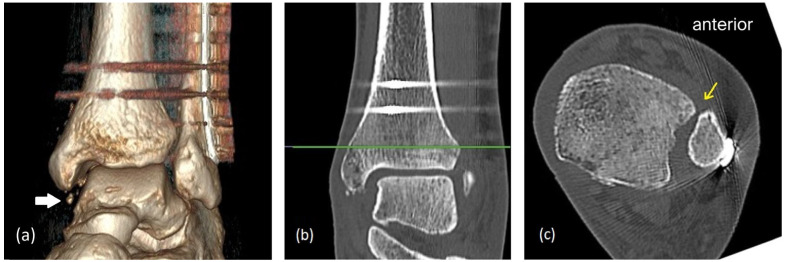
Postop CT scan showing avulsed fragments in the medial gutter (solid white arrow, (a)); anterior syndesmotic malreduction (yellow arrow, (c)), referenced at 1 cm proximal to the ankle joint line (green line, (b)).
